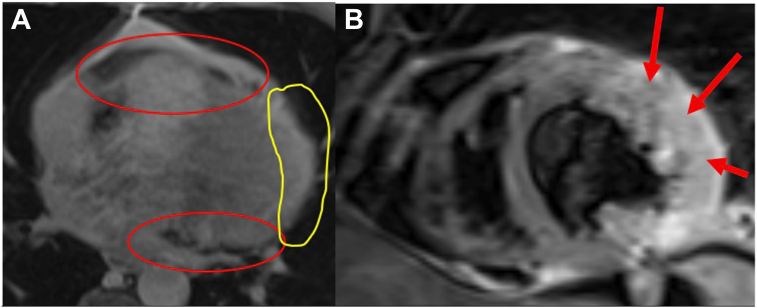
(A) CMR, axial postcontrast CAIPIRINHA (controlled aliasing in parallel imaging results in higher acceleration)–accelerated VIBE (volumetric interpolated breath-hold examination) with fat suppression in the delayed phase, demonstrates absence of the pericardium (*yellow oval*) along the lateral wall; remaining pericardium is visualized (*red ovals*). (B) CMR, short-axis T2-weighted triple inversion recovery sequence in our patient, demonstrates myocardial edema of the herniated wall (*red arrows*).
